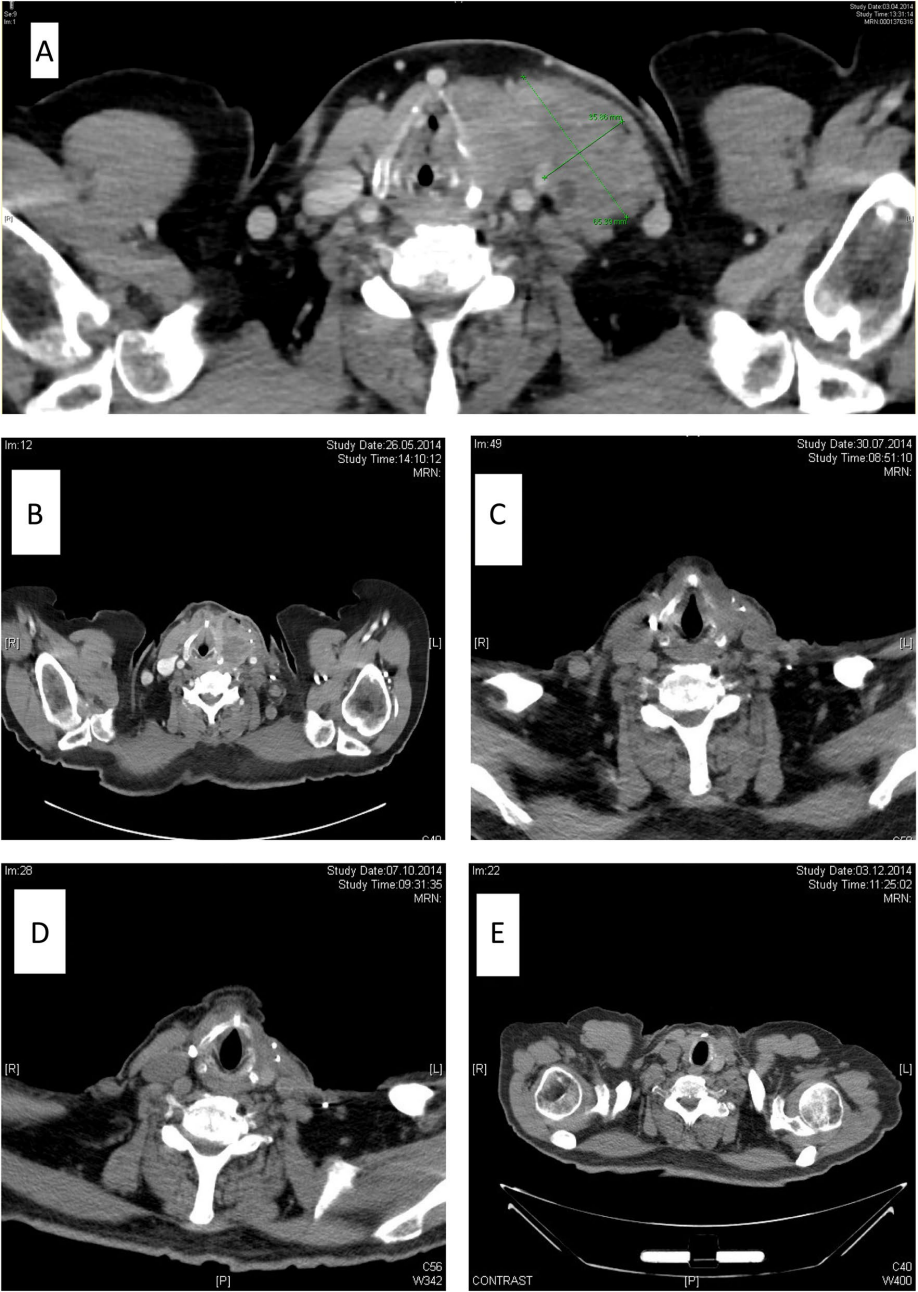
CT-scan, left sided thyroid tumor; A initial situation, tumor size 65 × 37 mm. B postoperative status on day 20; tumor size 44 × 26 mm. C status 2 months after initiation of vemurafenib; tumor size 35 × 22 mm. D status 5 months after initiation of vemurafenib; tumor size 33 × 16 mm. E status 7 months after initiation of vemurafenib; tumor size 33 × 16 mm
